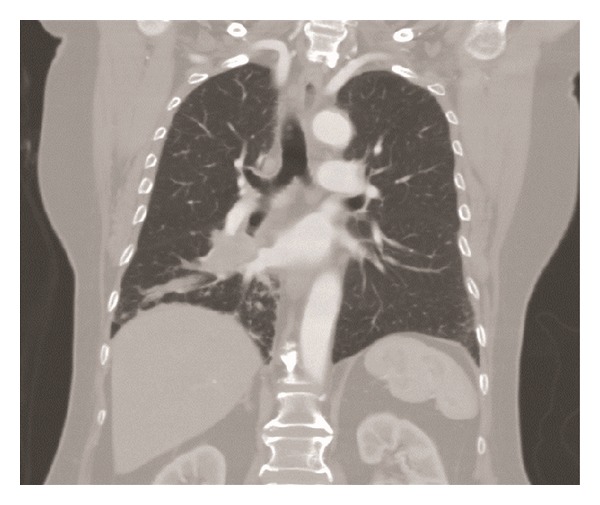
Transverse section on a chest CT demonstrating the presence of tumor lesions in the right middle and lower lobes.
